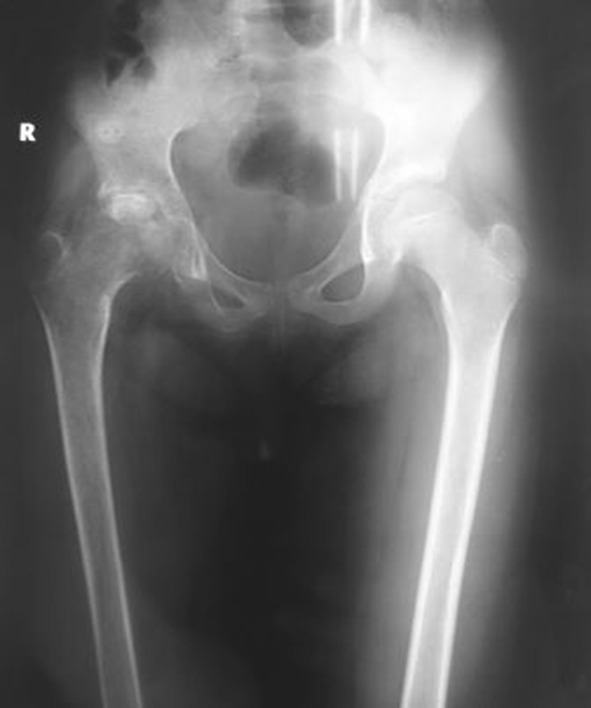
Plain radiograph of the pelvis with both hips of a 10-year-old with right side hip joint tuberculosis (clinico-radiological stage 2), which was treated with arthroscopic debridement
